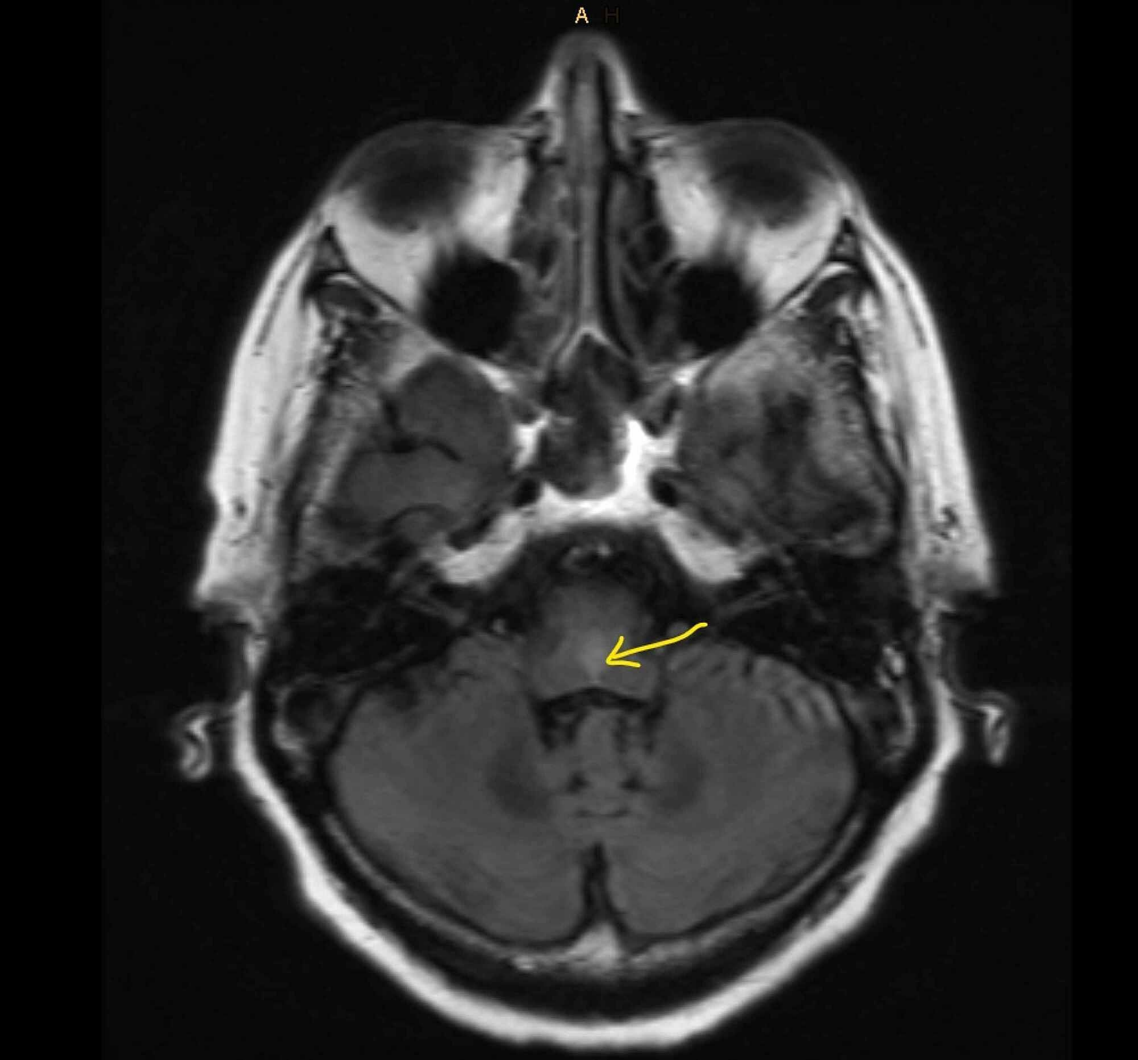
T2 FLAIR before treatment. FLAIR, fluid-attenuated inversion recovery
